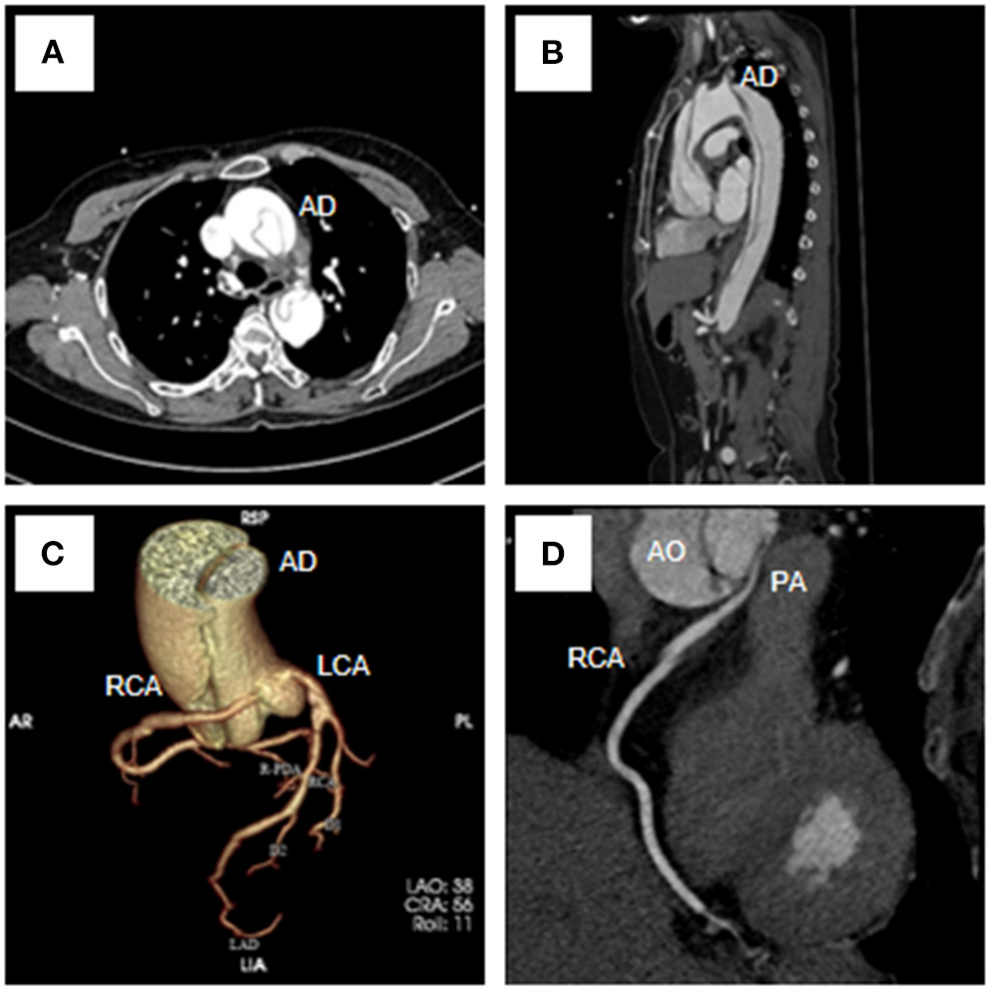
Total aorta plus coronary computed tomography angiogram showed the Stanford type A aortic dissection (A–C), the RCA originated from the LCS (C,D) and coursed between the aorta and the pulmonary artery (D). RCA, right coronary artery; LCA, left coronary artery; AD, aortic dissection; AO, aortic; PA, pulmonary artery.
